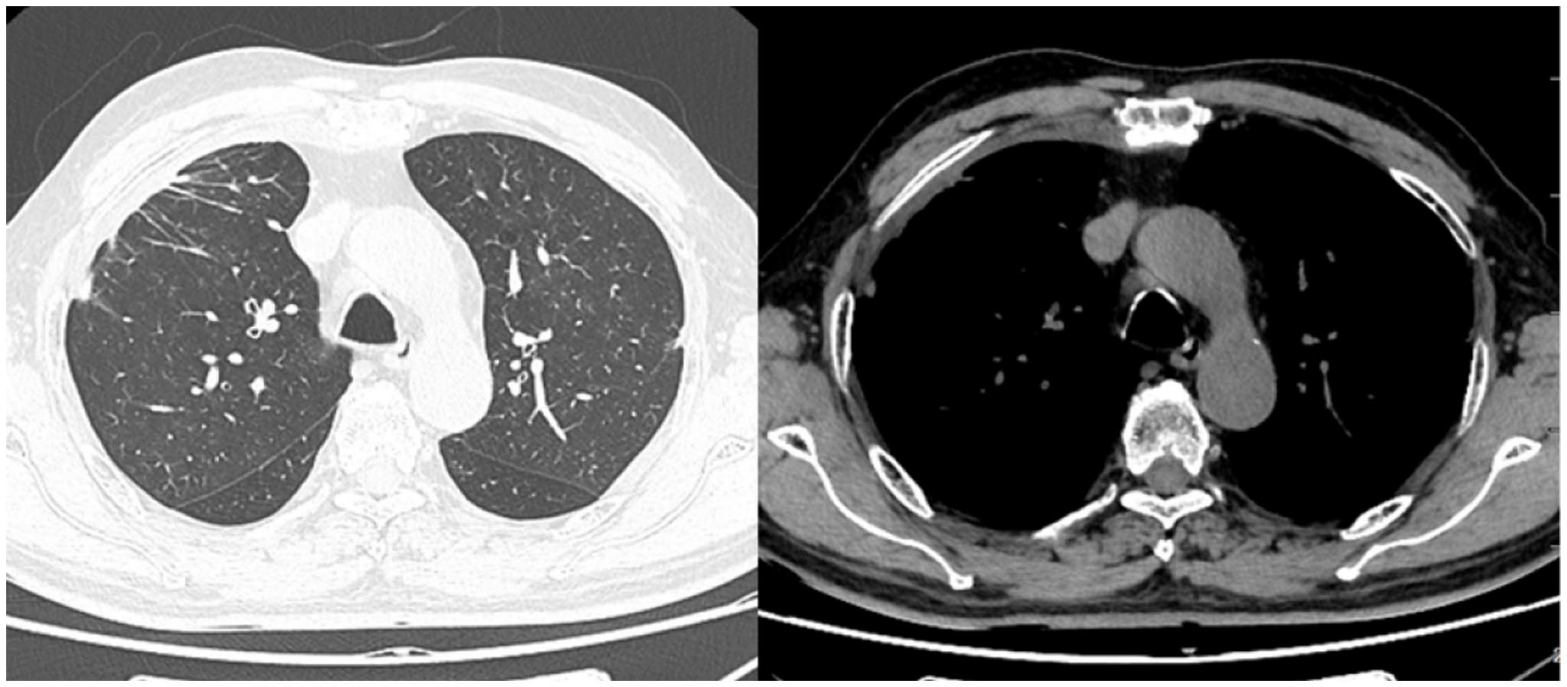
Chest CT after treatment: patchy lesions and pleural effusion in the right lung almost disappeared.
